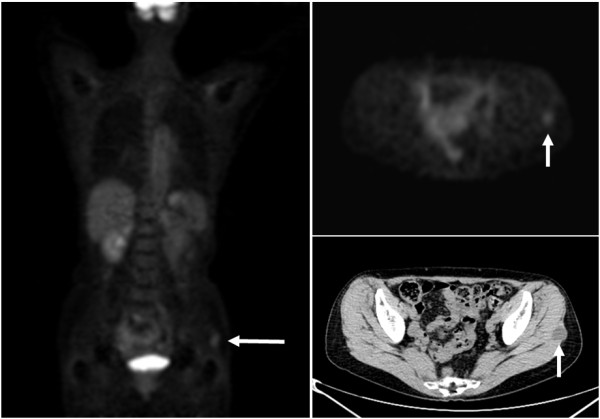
FDG PET images demonstrate an increased uptake in the left buttock. Transaxial CT shows a 3.5 cm hypodense mass, with corresponding tracer uptake (arrows).
